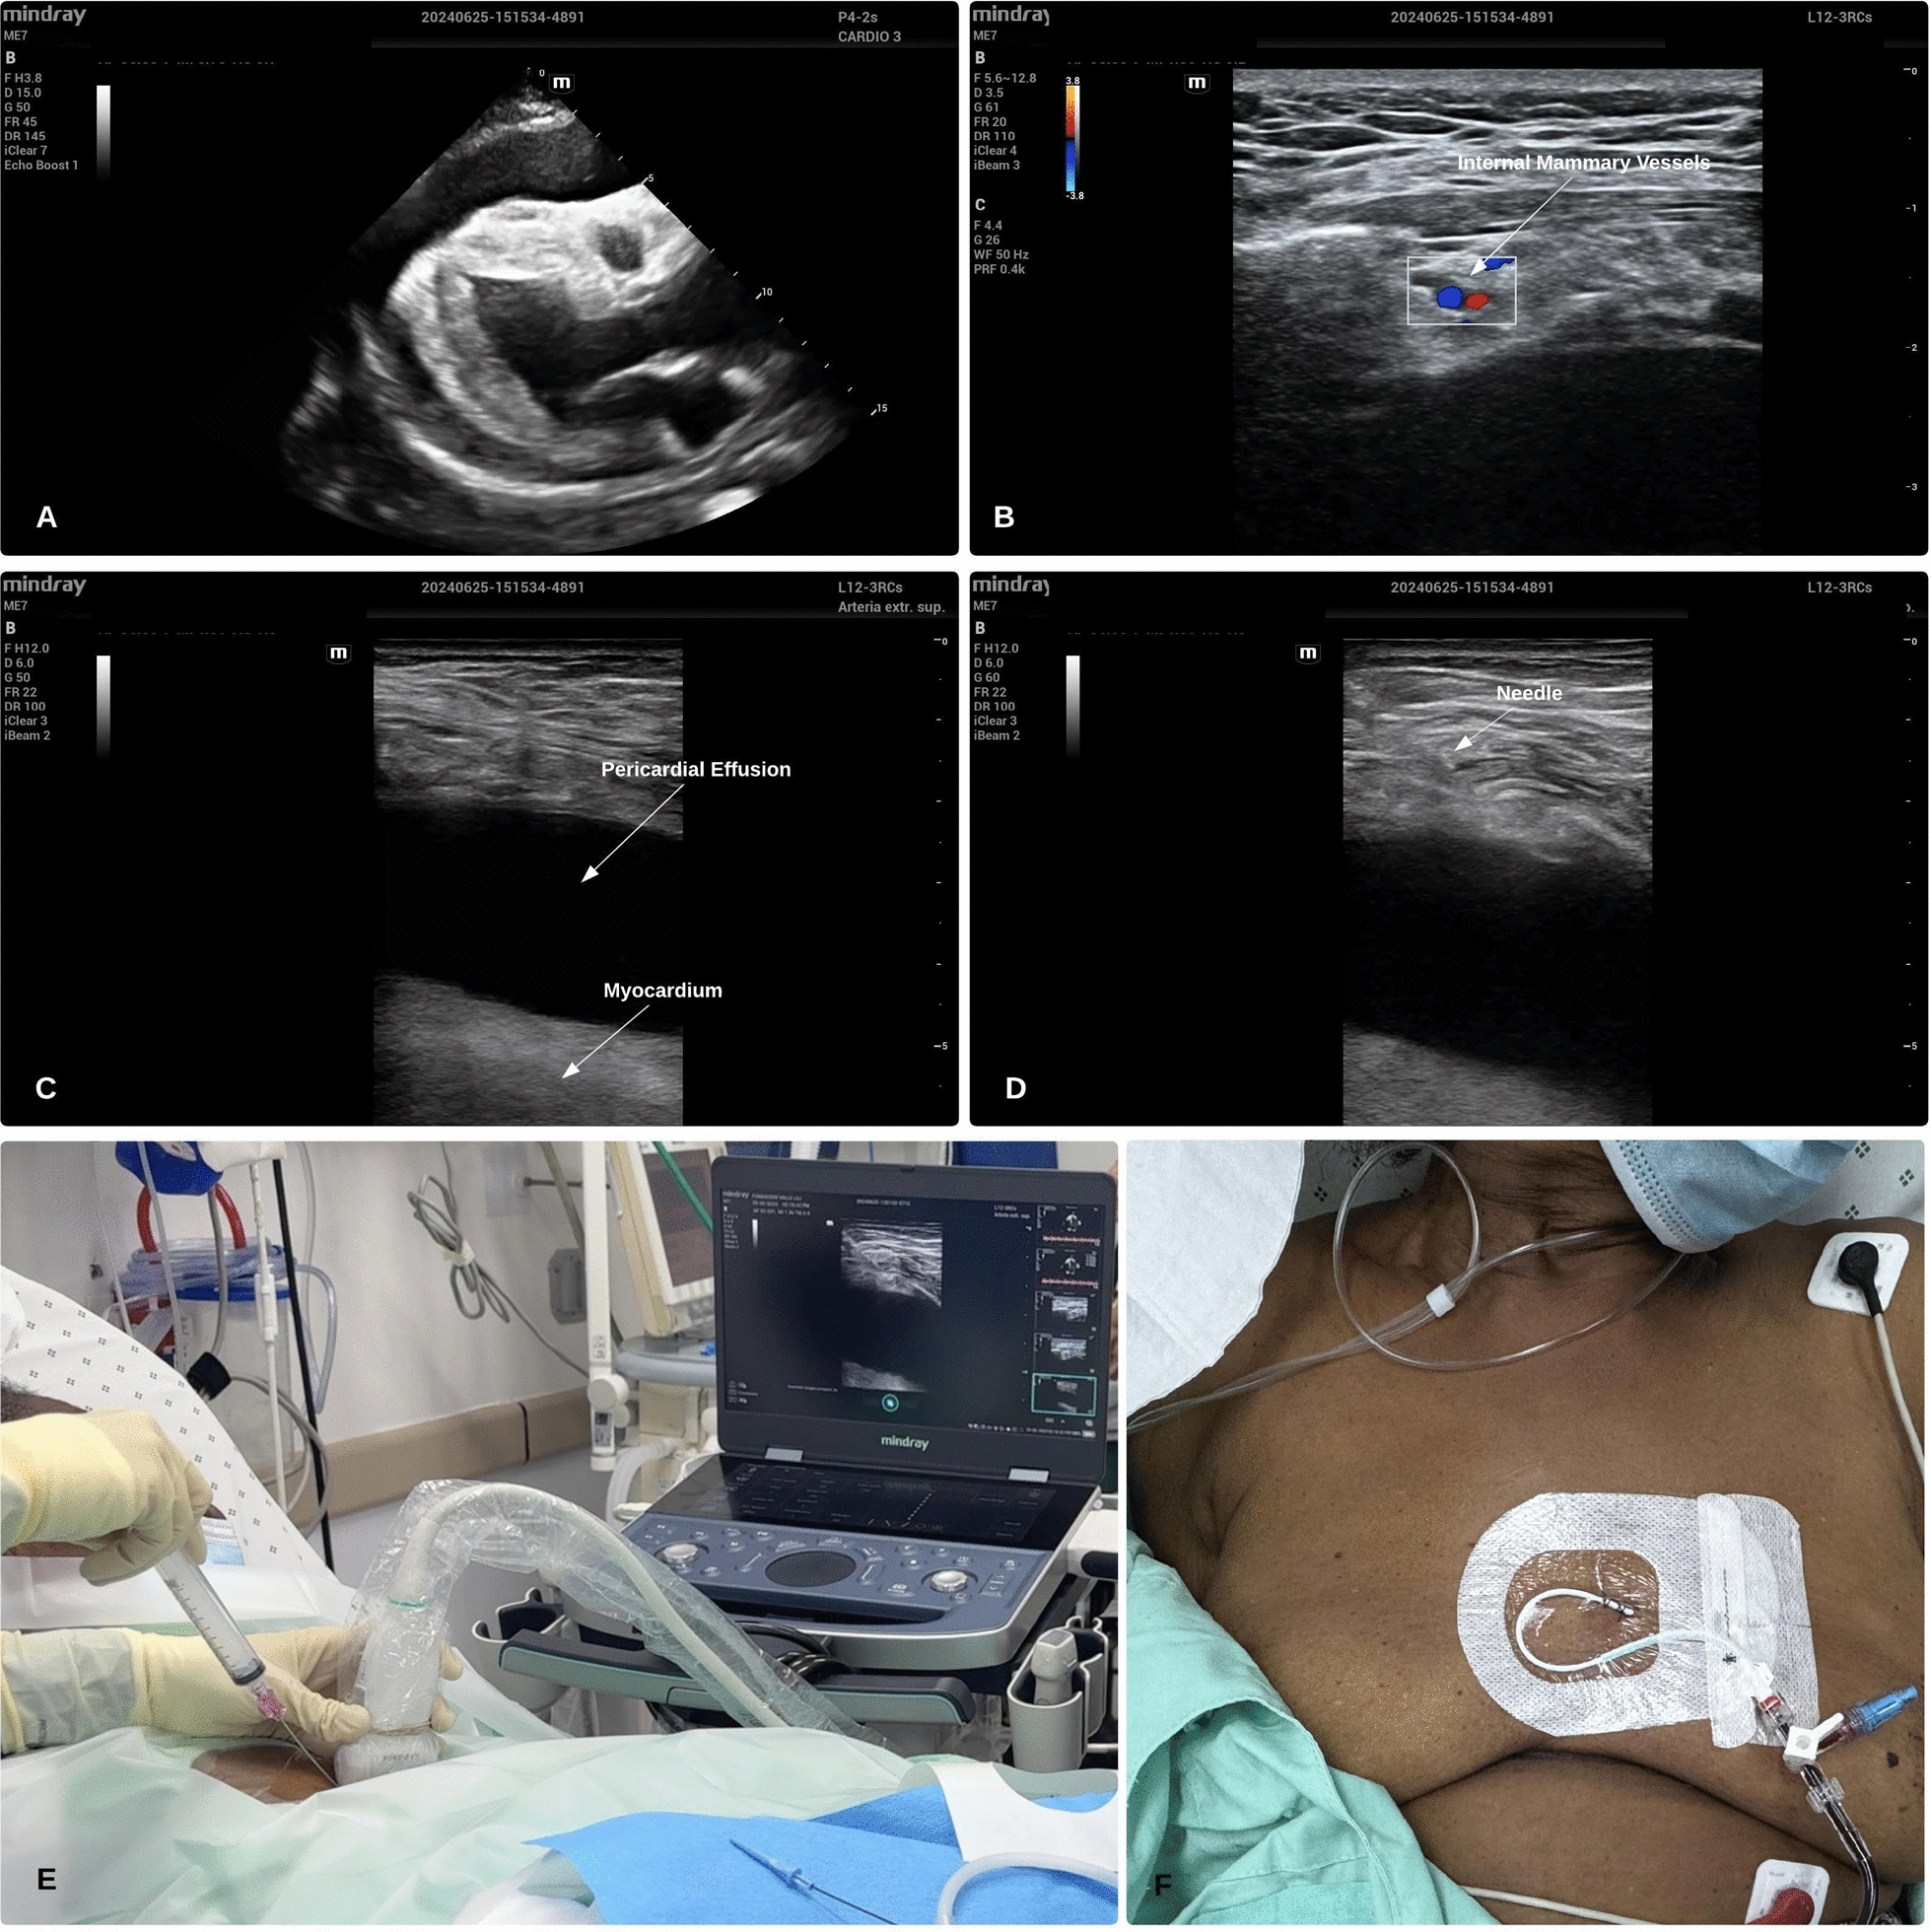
Real-time, in-plane US-guided pericardiocentesis. A phased-array probe is used to locate the largest pericardial fluid accumulation in the parasternal o para-apical windows a. A high frequency linear probe is used to locate the internal thoracic vessels b, to estimate the distance from the skin to the pericardial sac c and to guide needle trajectory using a real-time in-plane technique, until the needle tip reaches the pericardial sac d, e. Catheter secured to the skin with a standard suture technique f
